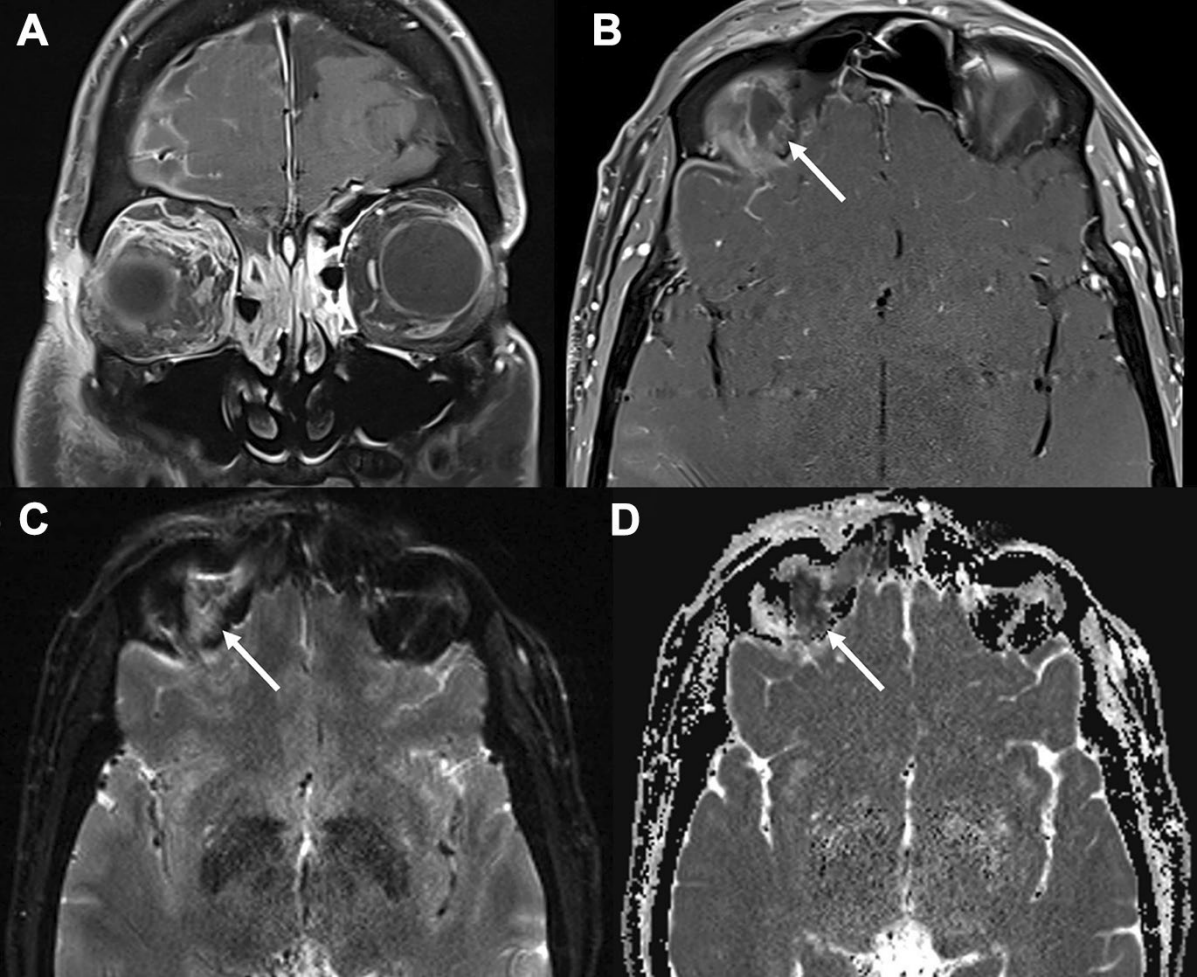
MR orbital imaging in Patient 4. A and B demonstrate T1 fat-suppressed contrast-enhanced MRI of the superior SPA (white arrow) with central non-enhancement indicative of an abscess. C and D demonstrate high signal (white arrow) on diffusion-weighted imaging and low signal on apparent diffusion coefficient (ADC) mapping exhibiting a low signal (white arrow), respectively, consistent with an abscess
